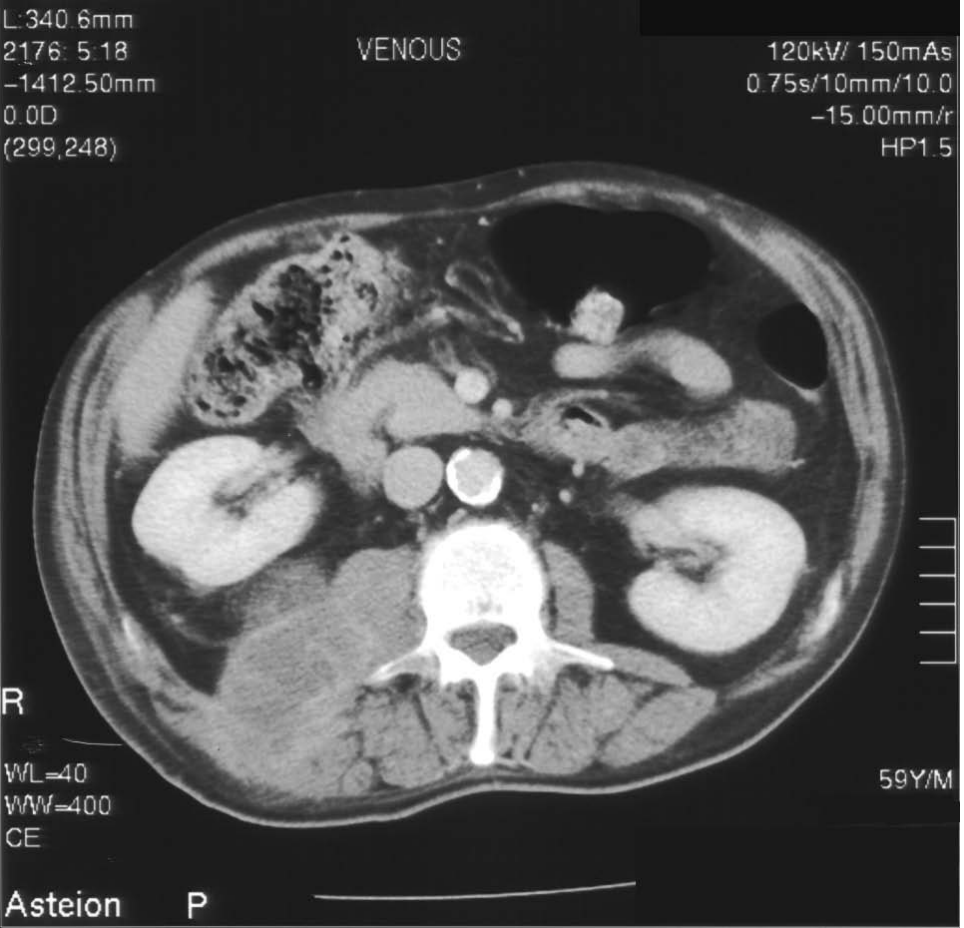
Computed tomogram of abdomen showing right psoas abscess extending from above the right kidney.
